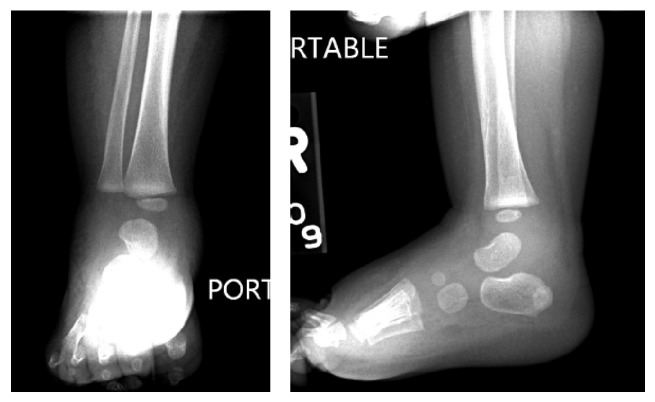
Radiograph of right ankle revealing moderate soft tissue swelling.
